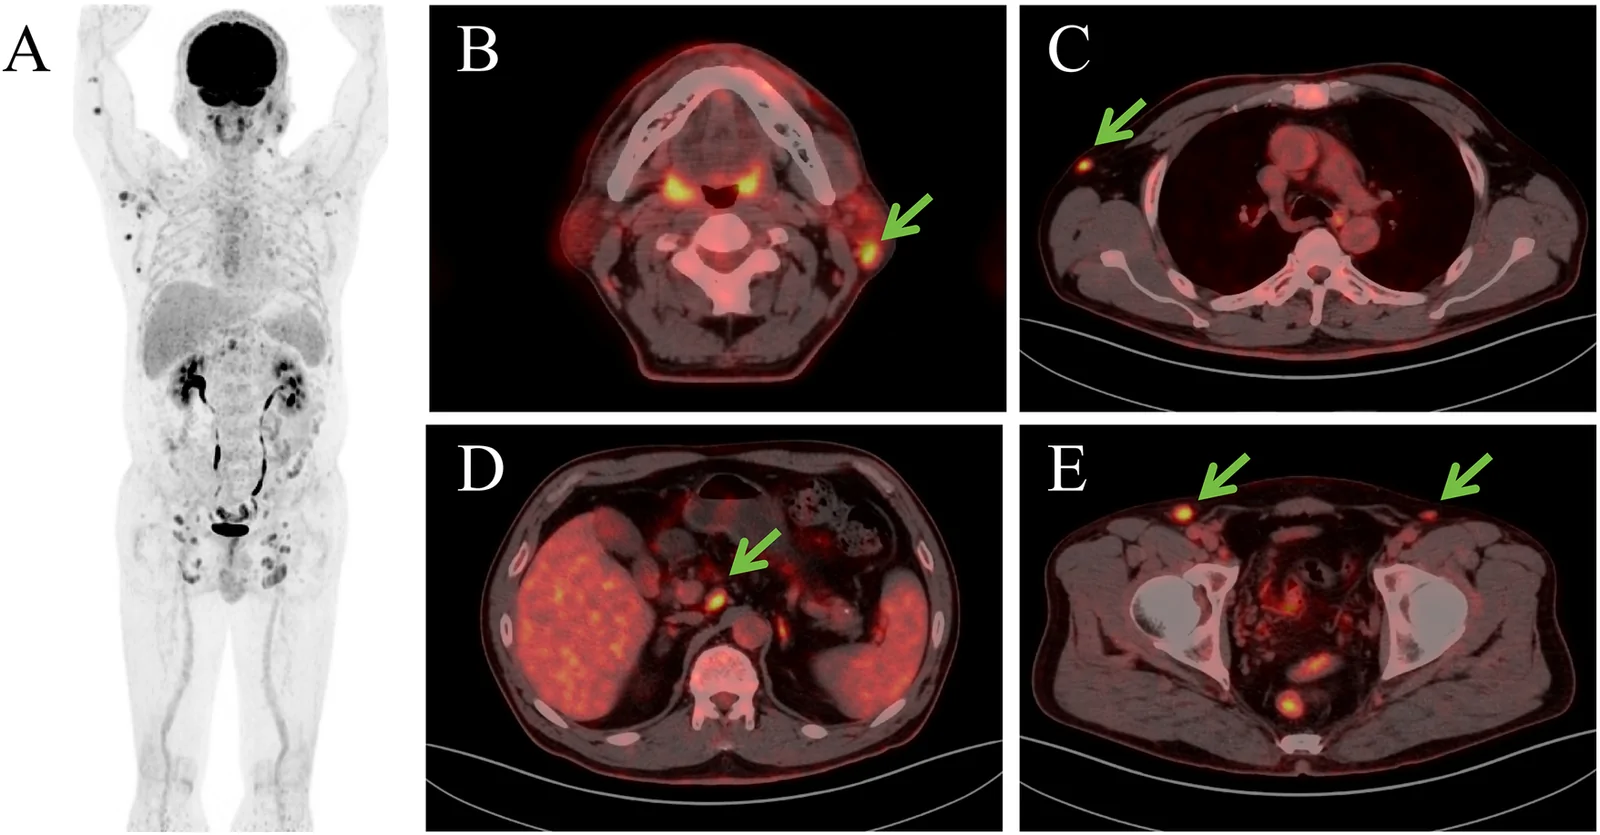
Whole-body ^18^F-FDG PET/CT imaging. (A) Maximum intensity projection (MIP) image demonstrating multisite hypermetabolic foci localized to the cervical, axillary, retroperitoneal, and inguinal regions. (B–E) Representative axial fused PET/CT images identifying intensely FDG-avid lymph nodes (arrows) in the left postauricular region (B), right axilla (C), para-aortic/retroperitoneal space (D), and bilaterally in the inguinal regions (E).
